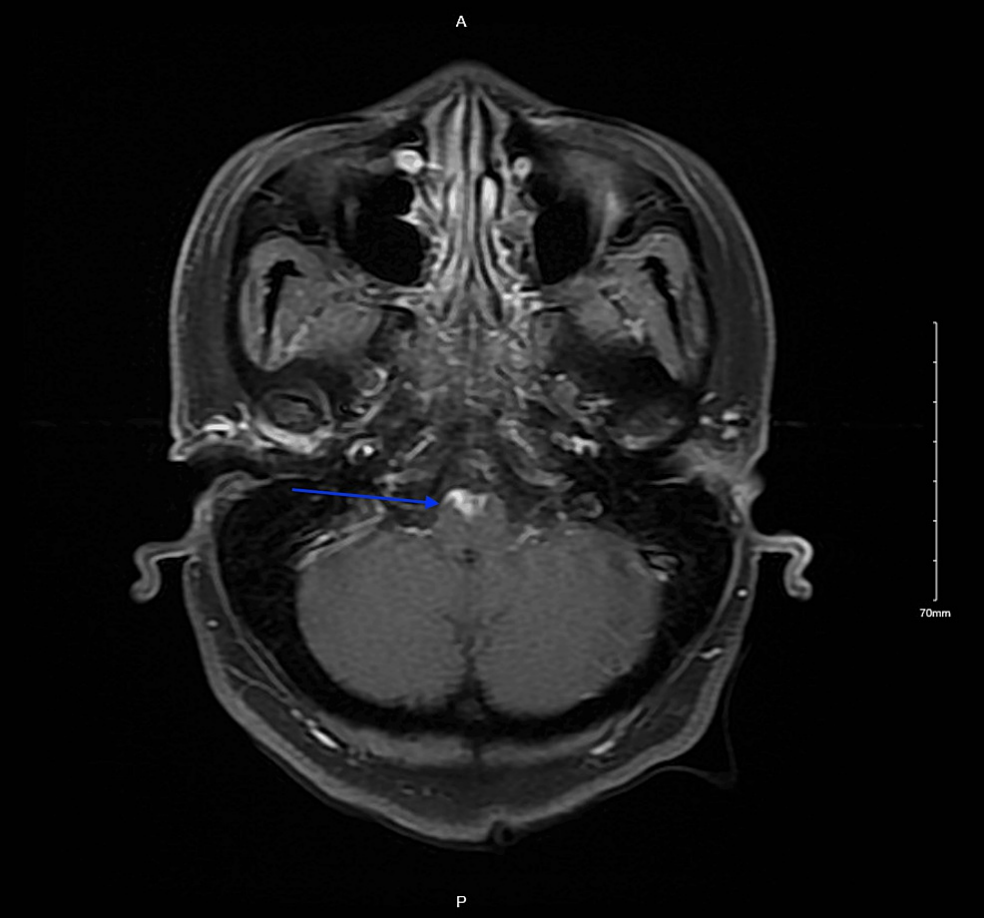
MRI brain with contrast showing anterior inferior pontine enhancement (blue arrow)
